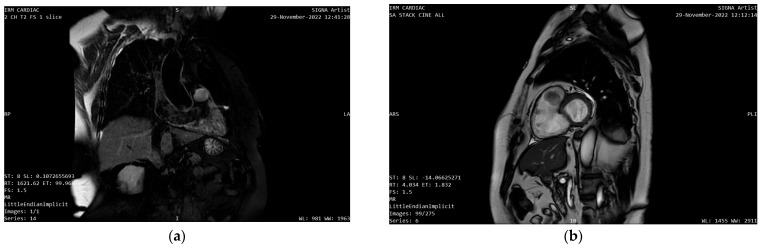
Cardiac MRI (CMR) showing the mass’ (white arrow): (a) high signal intensity on the fat suppressed T2 weighted sequence; (b) late contrast enhancement.
